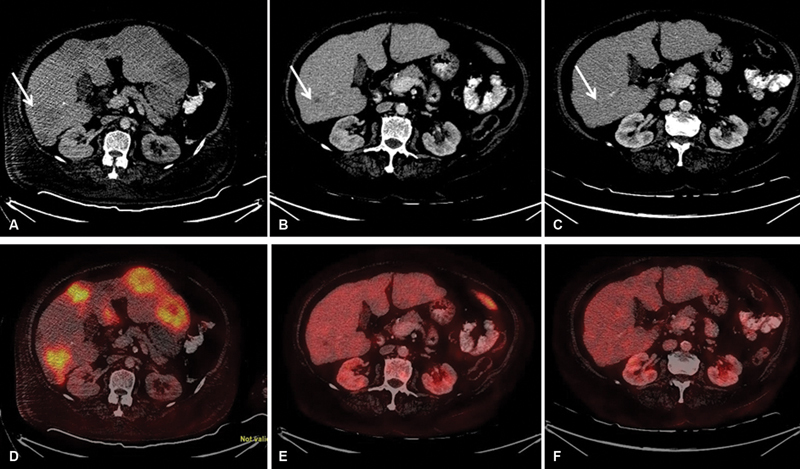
Transaxial contrast-enhanced computed tomography and positron emission tomography-computed tomographic images at baseline (
